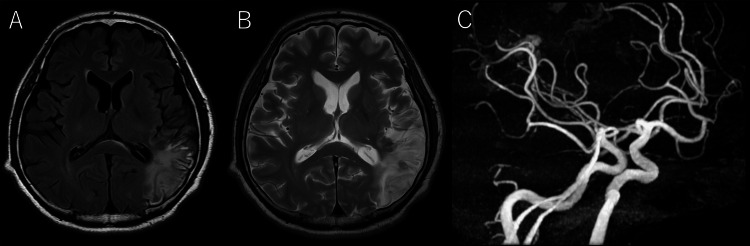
Post-coil embolization head MRI/MRA. (A) FLAIR image and (B) T2-weighted image reveal no new cerebral infarcts. (C) MRA confirms the complete obliteration of the aneurysm. MRA: Magnetic Resonance Angiography; FLAIR: Fluid-Attenuated Inversion Recovery image.
